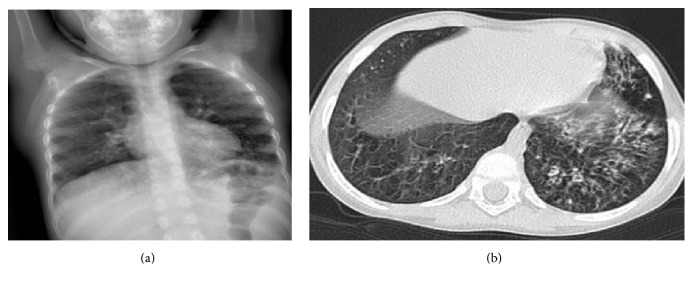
(a) Chest X-ray: bilateral infiltrations, bronchial thickenings, and air trapping. (b) Thorax CT: bronchiectasis in the left upper lobe and lower lobe, peribronchial thickenings, mucous plaques, and air trapping in both lungs (At four years of age).
